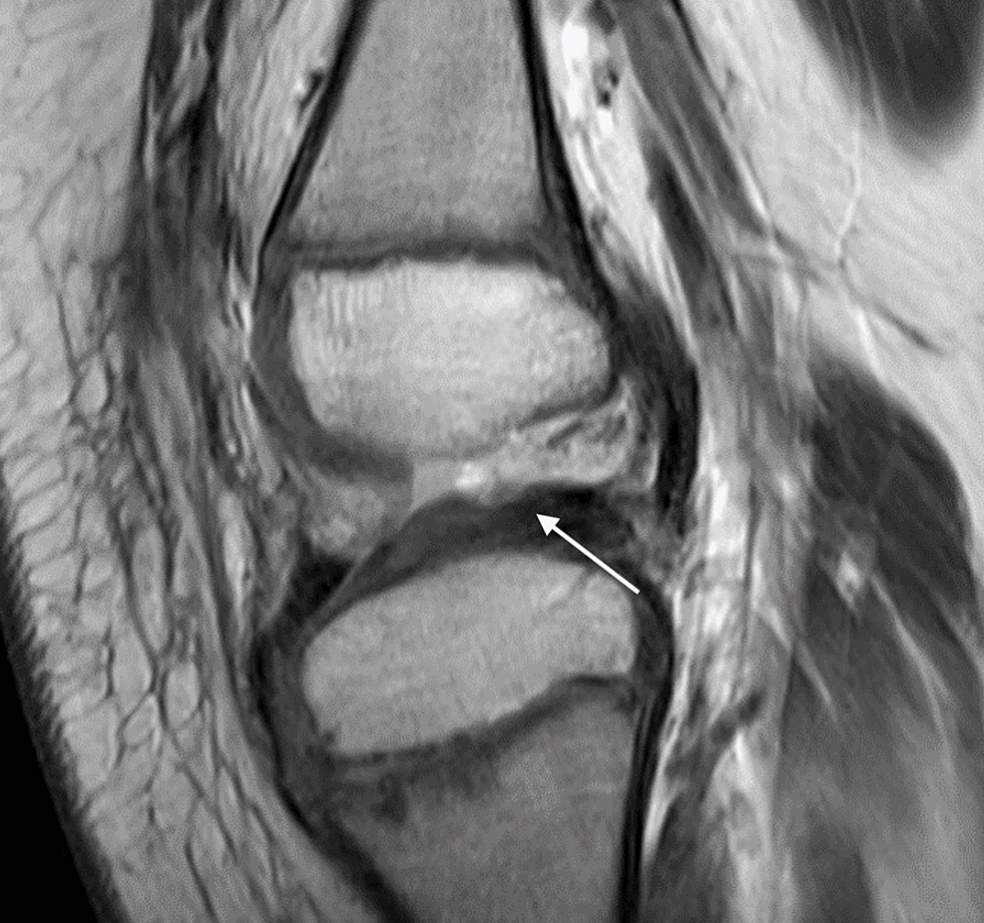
Lateral View of Right Knee MRI of Patient 2 Note the absence of the anterior cruciate ligament (white arrow) and anterior tibial translation
